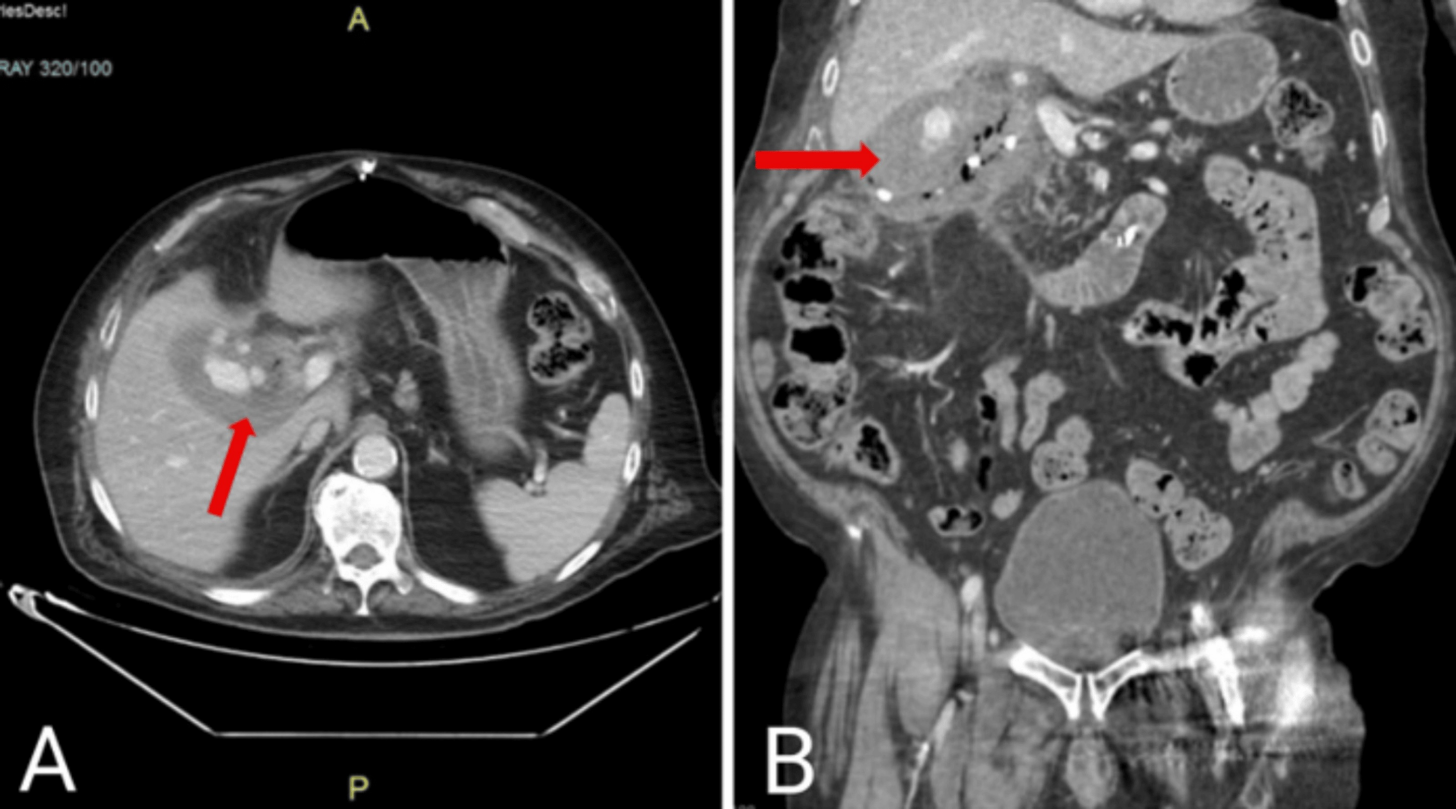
CT angiogram with IV contrast showing diffusely emphysematous gallbladder with edematous wall and patchy enhancement (red arrows) pattern consistent with cholecystitis (A) Axial. (B) Coronal.
